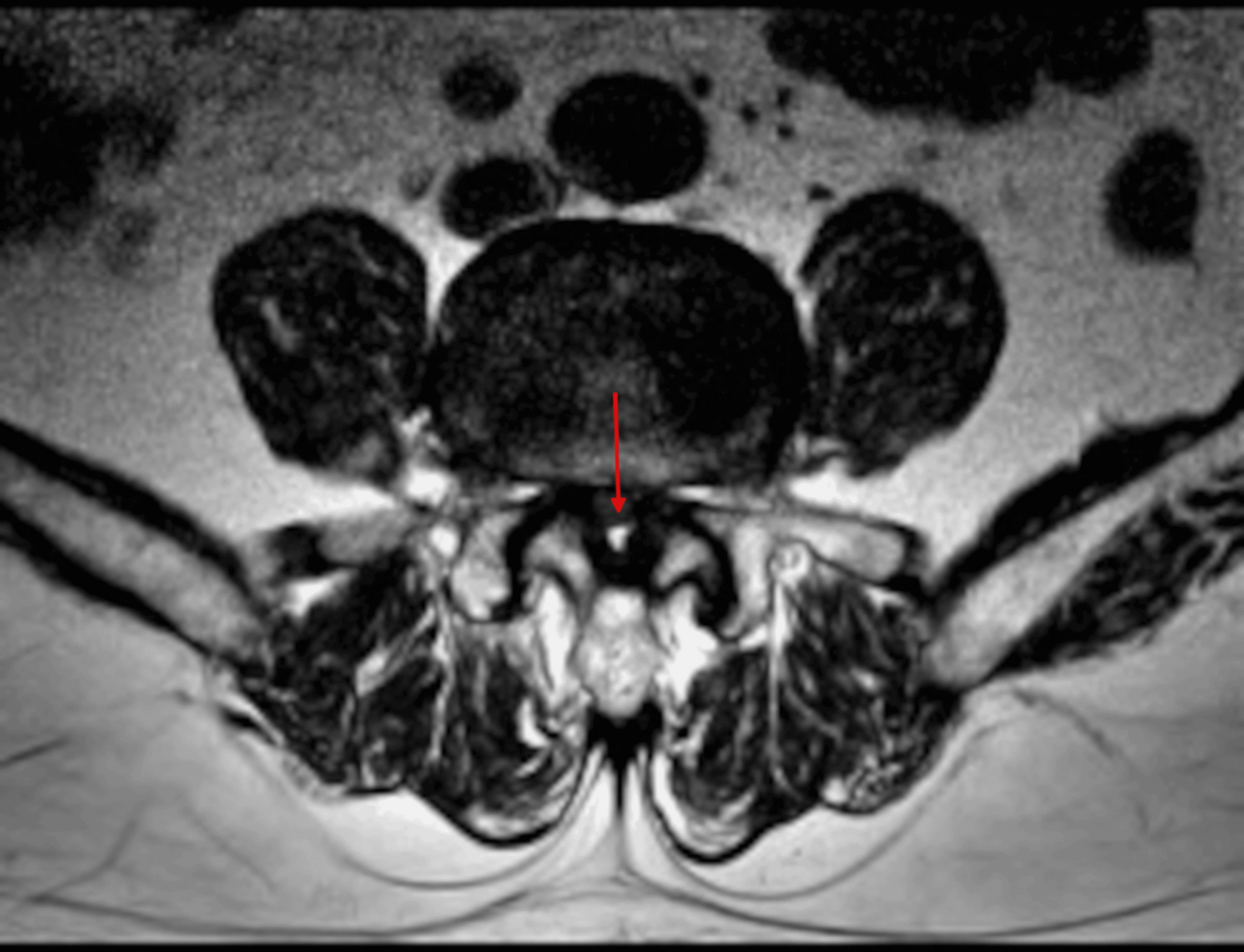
Lumbar spine MRI (T2), axial view before the procedure. The red arrow indicates severe central canal stenosis at L4/5. Axial T2-weighted MRI of the lumbar spine demonstrating markedly narrowed central spinal canal at the L4/5 level, as indicated by the red arrow.
